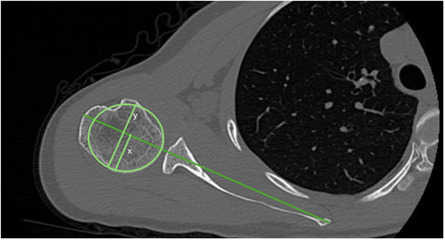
Axial computed tomography scan of the shoulder demonstrating the method for calculating the Posterior Subluxation Index (PSI) using Friedman's line. Measurement Y represents the anteroposterior diameter of the humeral head, and measurement X represents the distance from the anterior margin of the humeral head to Friedman's line. The PSI is calculated as (X/Y) × 100. In this case, the PSI was approximately 68.8%, indicating significant posterior humeral head subluxation.
